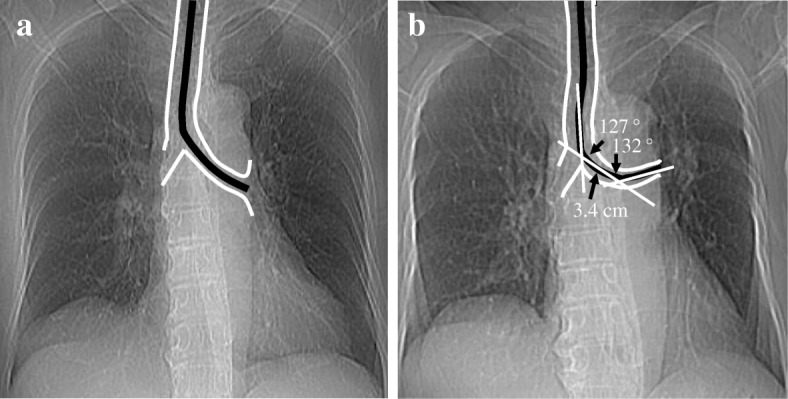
Chest X-rays before the previous lingula-sparing left upper lobectomy (a) and before the current robot-assisted right upper lobectomy (b). An outline and a centerline of the trachea and the left main bronchus are delineated with white and black lines, respectively, on each chest X-ray. The distance from the trachea to the angulation point is 3.4 cm. Note that compared with the chest X-ray a, the chest X-ray b exhibits mild angulation of the left main bronchus, indicated by a combination of the 127° T-MB angle and the 132° PL-DL angle, which does not meet the criteria for remarkable bronchial angulation, defined by a combination of a wide T-MB angle (> 140°) and a narrow PL-DL angle (< 100°) [6]. T-MB angle, angle formed by the trachea and left main bronchus; PL-DL angle, angle formed by the proximal and distal portions of the left main bronchus
